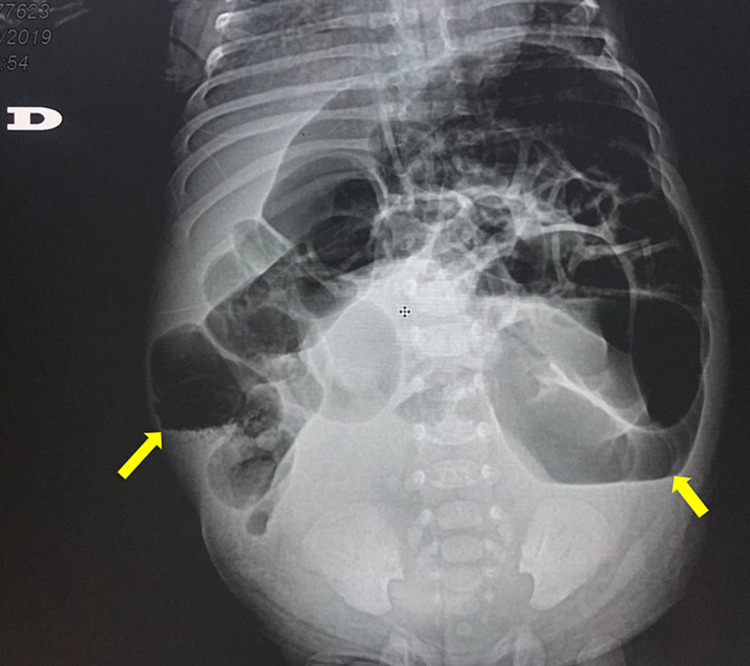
Patient's abdominal CT scan with evidence of small bowel distension
